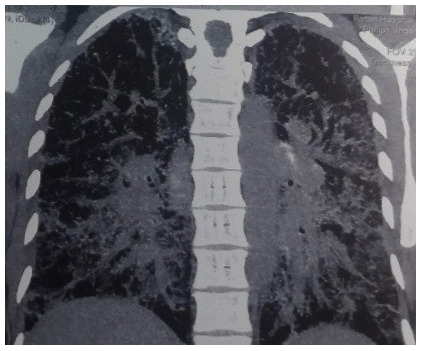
High-resolution computed tomography (HRCT) chest showing diffuse ground glass changes with interlobular septal thickening with mosaic attenuation and multiple enlarged calcified mediastinal and hilar lymph nodes.
